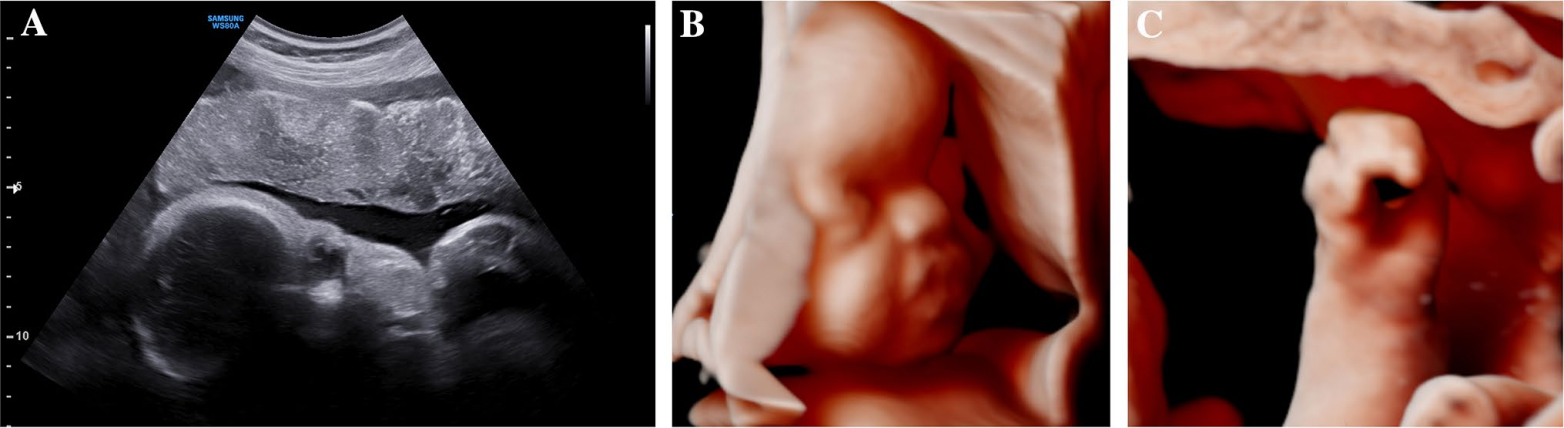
Fetus with Apert syndrome in 30 + 3 weeks of gestation. The ultrasound examination shows a prominent forehead with frontal bossing (a, b). Bilateral syndactyly can be imaged (c)
